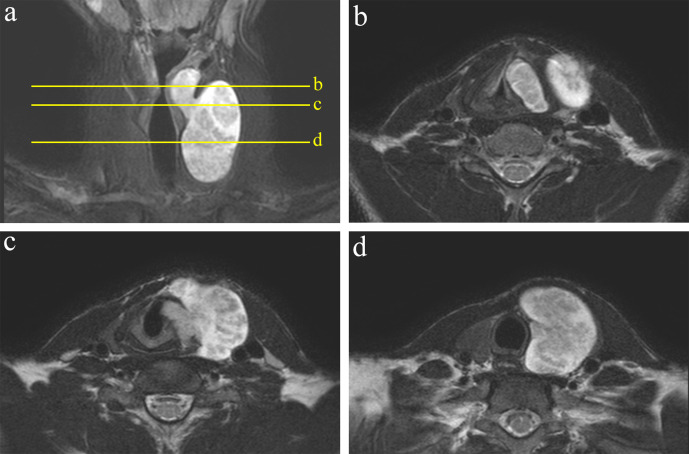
Magnetic resonance images. (a) Coronal fat-suppressed T2-weighted image shows
